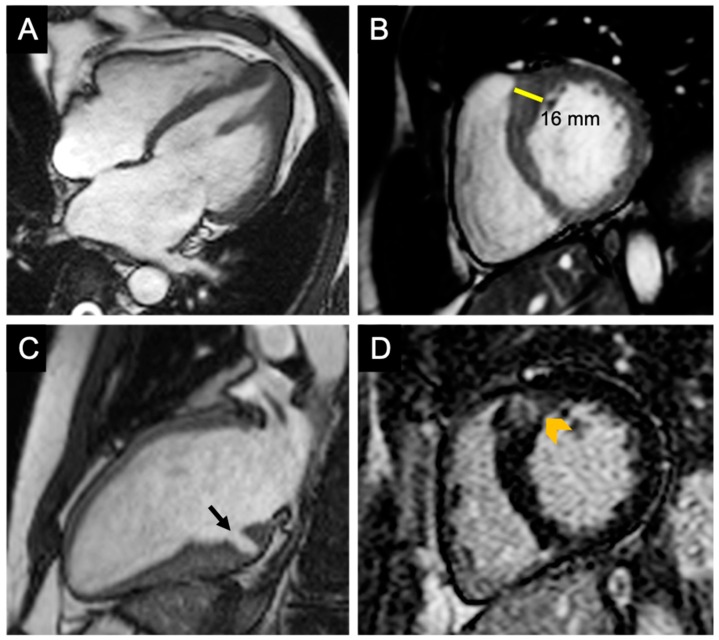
The role of CMR in the assessment of HCM. (A) Cine-CMR four-chamber view—diastolic phase demonstrating hypertrophy of the apical segments of the left ventricle and apical insertion of the papillary muscle in a patient with apical HCM. (B) Cine-CMR short-axis view—diastolic phase demonstrating hypertrophy of the confluence of the basal anterior septum with the contiguous anterior free wall; this is the most common location of LV hypertrophy in patients with HCM. (C) Cine-CMR apical two-chamber view—diastolic phase showing a myocardial crypt (black arrow) located in the basal inferior wall in a patient without definite criteria for HCM (the maximum wall thickness was 13 mm). (D) LGE short-axis view of the patient in panel B, demonstrating patchy hyperenhancement of the hypertrophied segment indicating replacement fibrosis. CMR—cardiovascular magnetic resonance, HCM—hypertrophic cardiomyopathy, LV—left ventricle, and LGE—late gadolinium enhancement.
